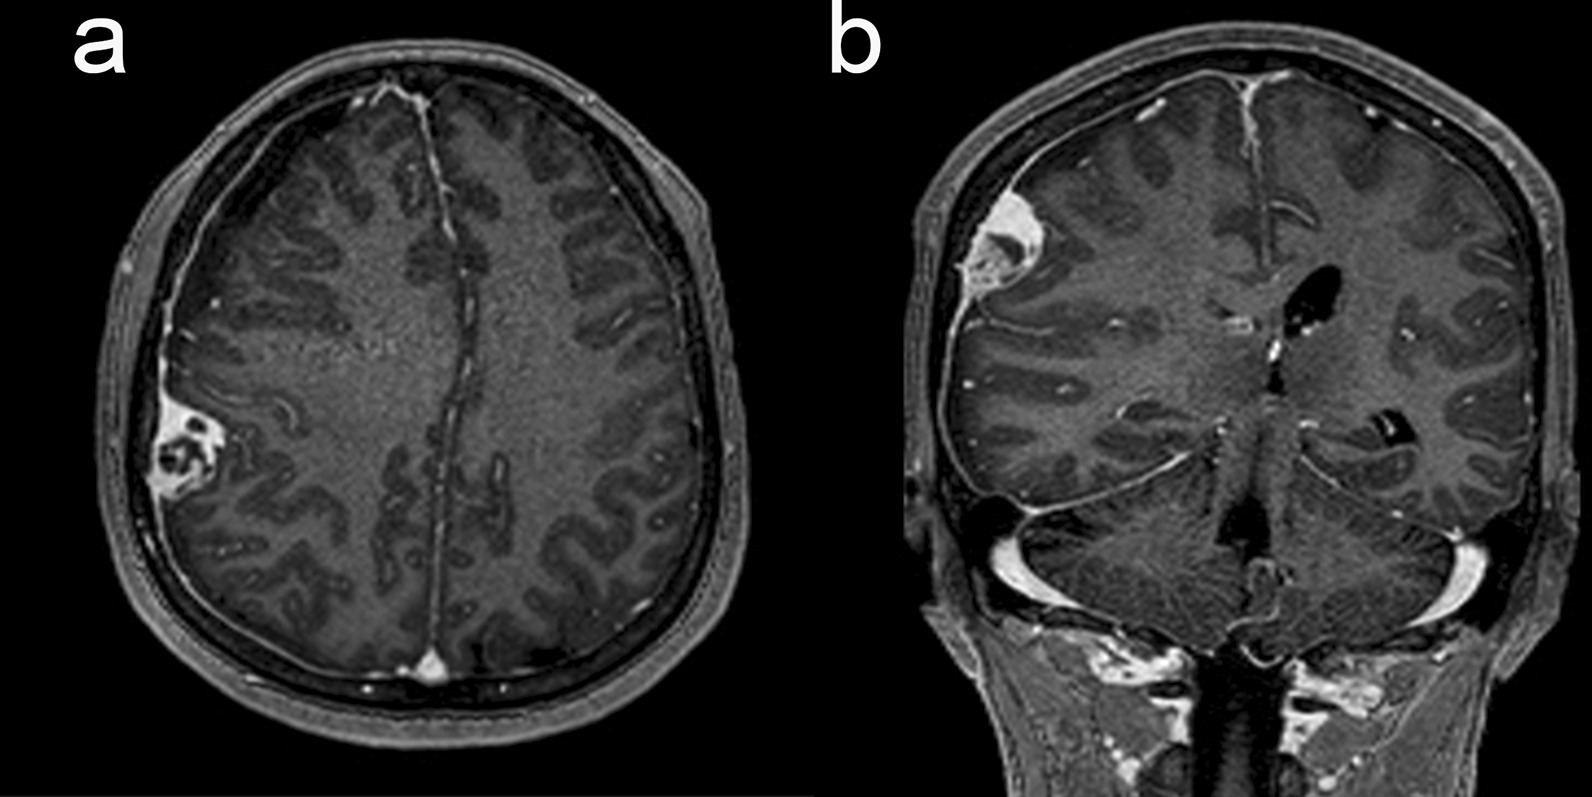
T1-weighted brain magnetic resonance images demonstrating a heterogeneously enhanced, 2.6 × 1.5 cm sized, extraaxial mass on the right parietal convexity with diffuse subdural hematoma in both axial view (a) and coronal view (b)
